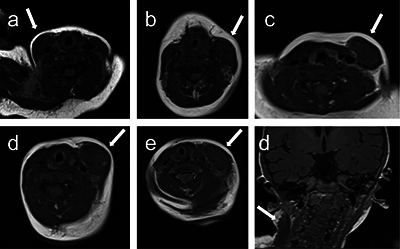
T1-weighted axial images from patients 1 to 5 (parts a–e) of the fibromatosis colli (arrows), isointense to the adjacent muscle. Part (d) is a T1-weighted coronal image of the fibromatosis colli (arrow) in patient 6.
